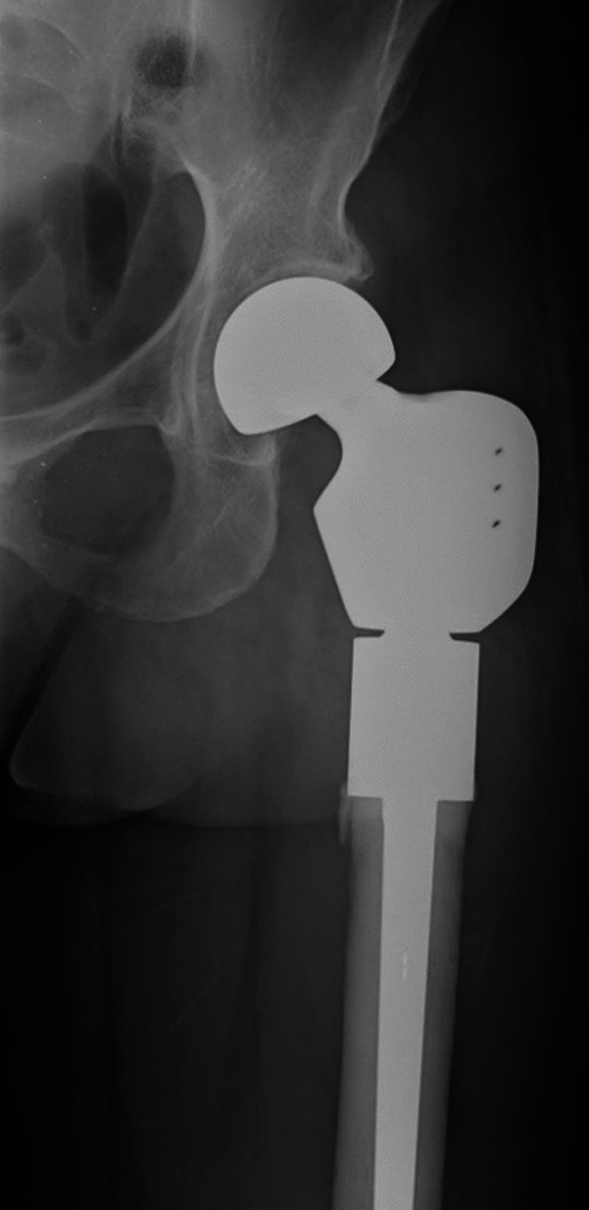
Post-operative X-Ray of a megaprosthesis of the proximal femur. Sites for the reattachment of the gluteal insertion are present in the trochanteric region of the implant
